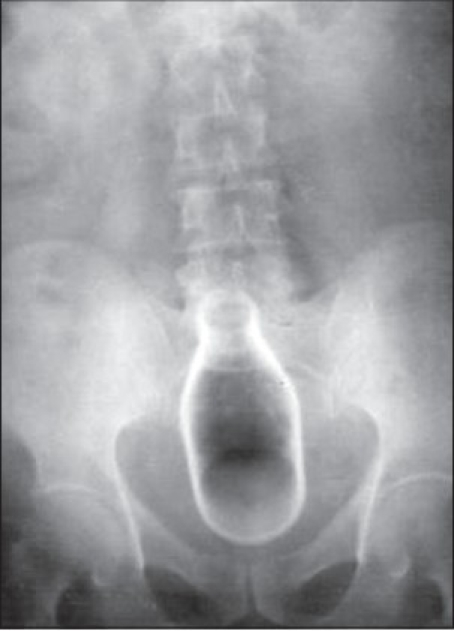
X-ray abdomen showing foreign body
